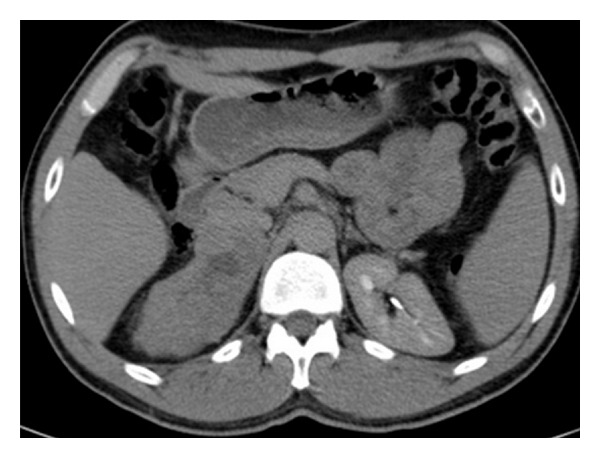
Axial CT at the delayed phase shows more or less homogenous enhancing with nonenhancing central scar tissue.
